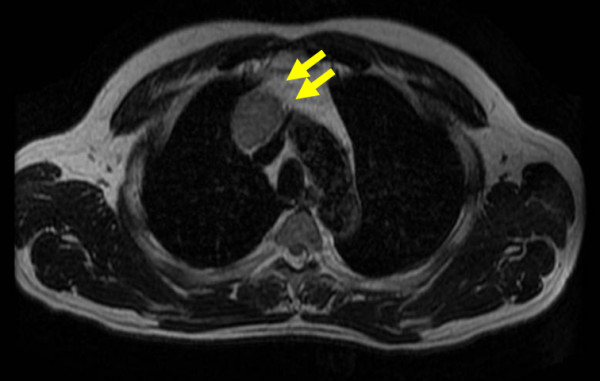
Chest MRI using intravenous contrast medium showed iso-intensity of the mass on a T2-weighted image with an unclear rim (arrows), as with the chest CT, too.
